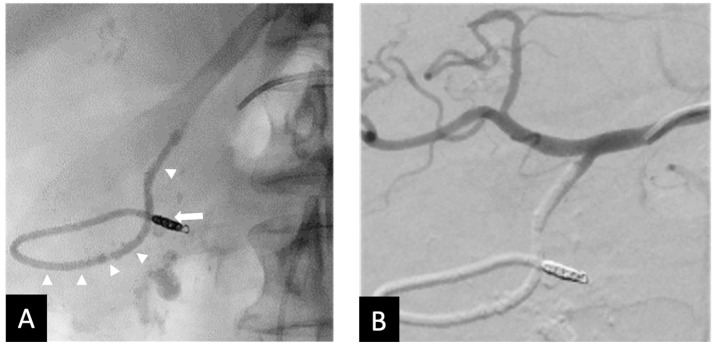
Seventy-three y.o. male with a large bleeding duodenal ulcer. Representative example of open-sandwich technique—(A) Post-embolization spot radiograph showing a 3 × 12 mm EmboldTM fibered coil (arrow) in the proximal right gastroepiploic artery (RGEA) and a continuous column of Ta-OCE (arrowhead) extending from the coil pack in the proximal RGEA to the proximal gastroduodenal artery (GDA) (B) Digital subtraction angiography (DSA) with contrast injection from the common hepatic artery (CHA) shows no flow in the GDA, RGEA, or any proximal branches, such as the supraduodenal artery (SDA). Image obtained from patient’s chart. Source: NK.
